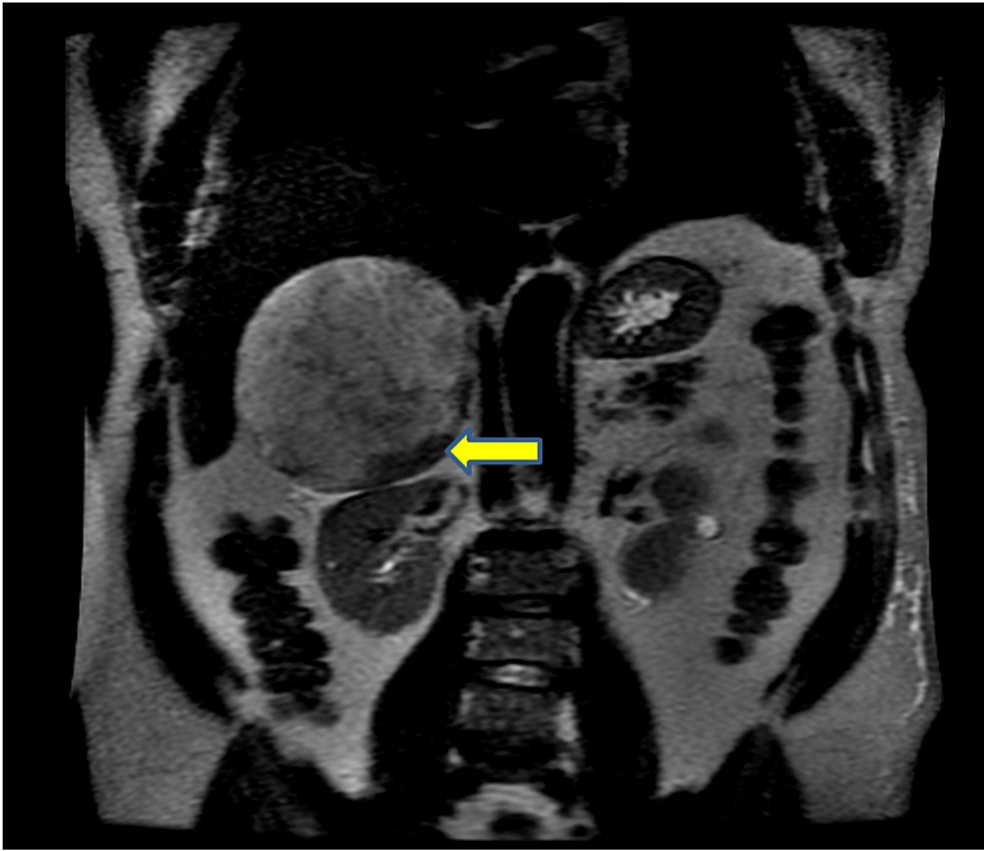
MRI T2 Non-Fat Saturated - Coronal View Image shows hemorrhage in the inferior aspect of the mass (yellow arrow).
